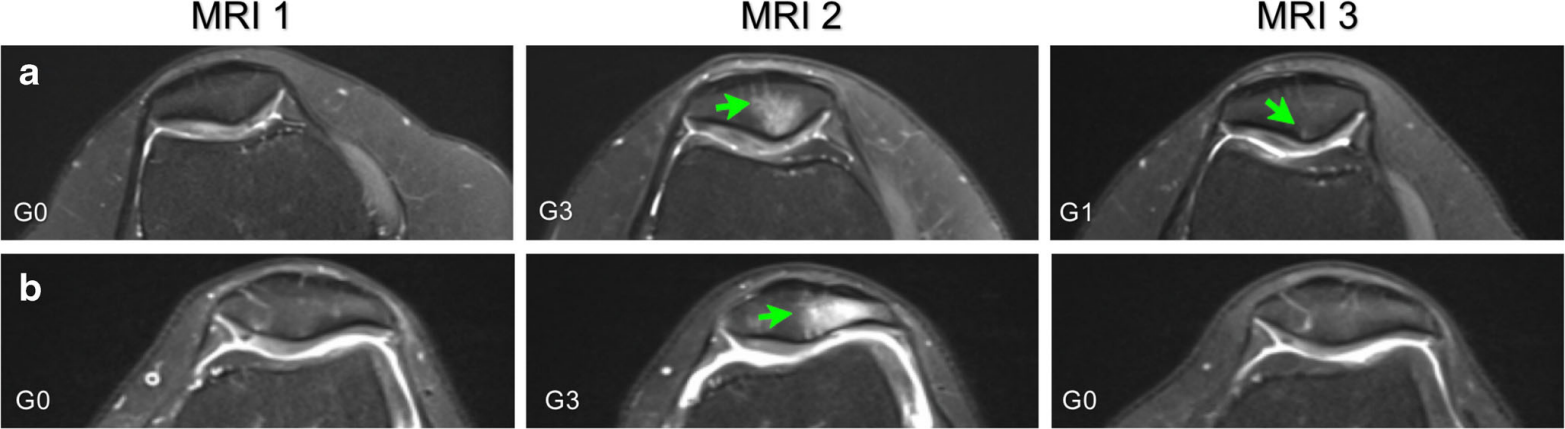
Axial proton-density fat-saturated MR images of two different knees that showed reversibility at 6-month follow-up (MRI 3) in the extent of subchondral bone marrow oedema-like signal of the patella that previously developed from the pre-marathon scan to the 2 weeks post-marathon scan (MRI 1 to MRI 2): A) reversibility but not to the MRI 1 grading status, in the right knee of a 31-year old woman; B) complete resolution to the MRI 1 grading status, in the left knee of a 34-year-old woman. Bone marrow oedema-like signal is indicated by arrows and the lesion grade (G) is included in the left bottom corner and is defined in the KOSS scoring system [18] (see Table 2); KOSS, Knee Osteoarthritis Scoring System
